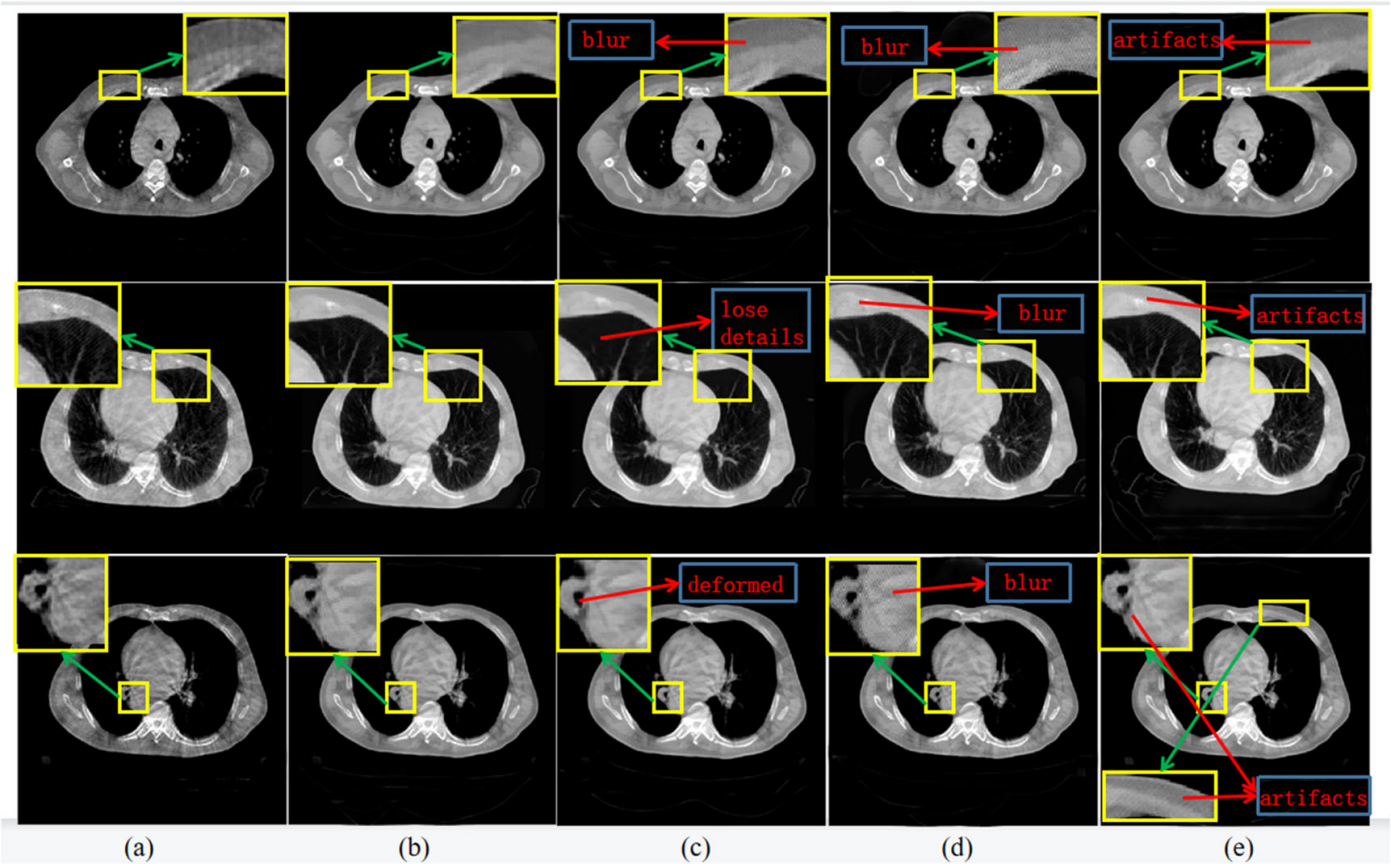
Artifact removal results obtained with chest data. (a) CBCT, (b) proposed correction, (c) method in Ref.,[26] (d) method in Ref.,[29] (e) method in Ref.[31] Row two display window [0,1528]. Other display window [498,1528].
